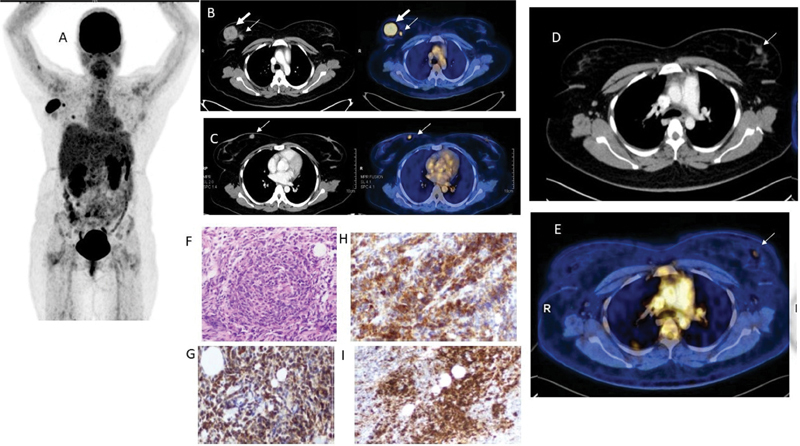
Follow-up FDG-PET/CT (fluorodeoxyglucose positron emission tomography/computed tomography) after 2 months showed complete resolution of all the hemophagocytic lymphohistiocytosis (HLH) lesions. New FDG avid multiple bilateral breast lesions (3 on right, 2 on left) were noted, (
